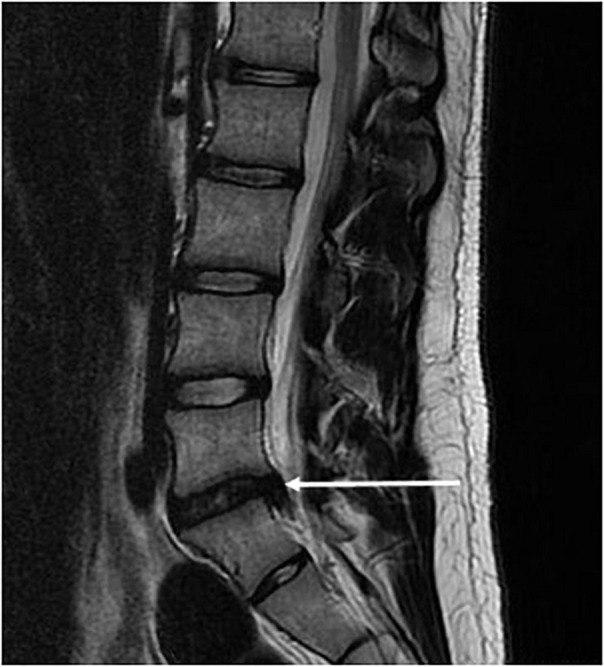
MRI of lumbar spine showing HNP, arrow added to original figure.
